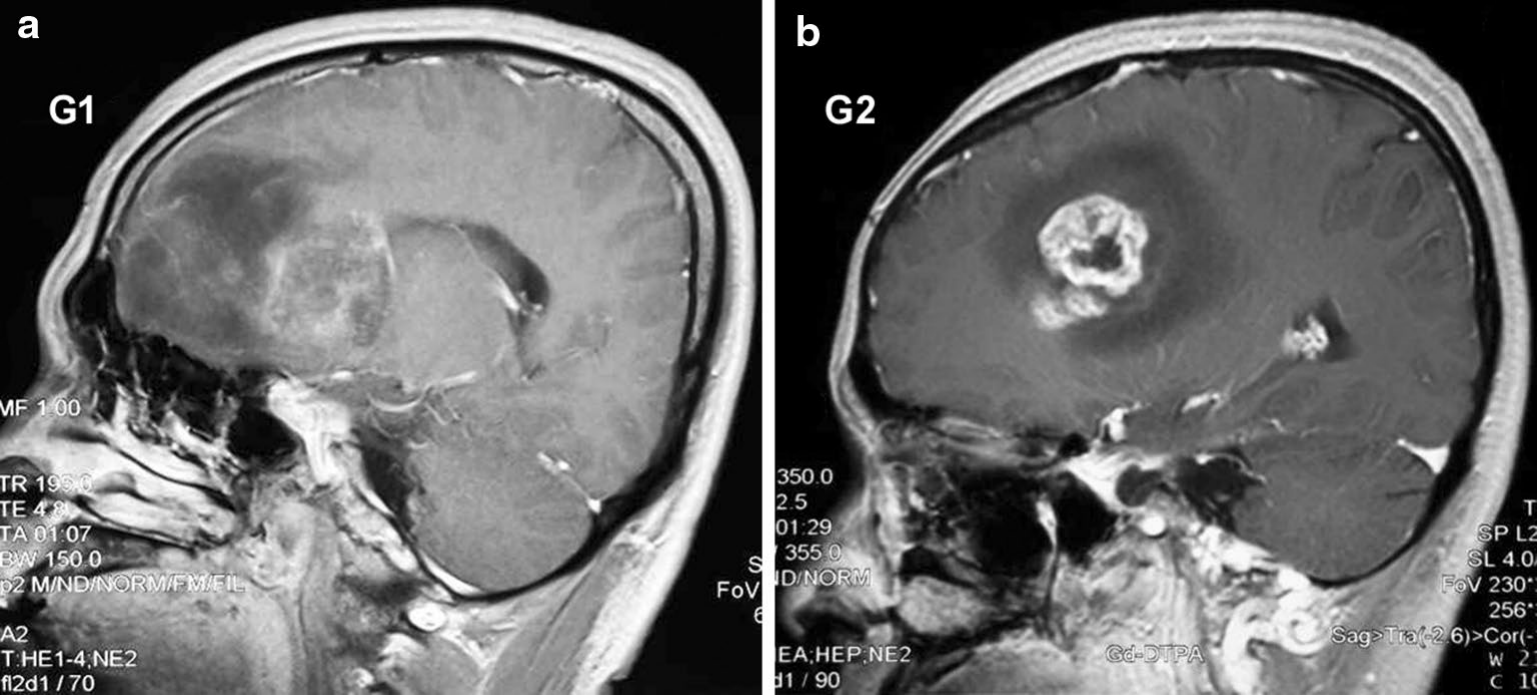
Head-enhanced MRI images for patients G1 and G2. a The head-enhanced MRI image of patient G1, who exhibited a glioblastoma in the *right* frontal lobe. b The head-enhanced MRI image of patient G2, who exhibited a glioblastoma in the *left* frontal lobe
